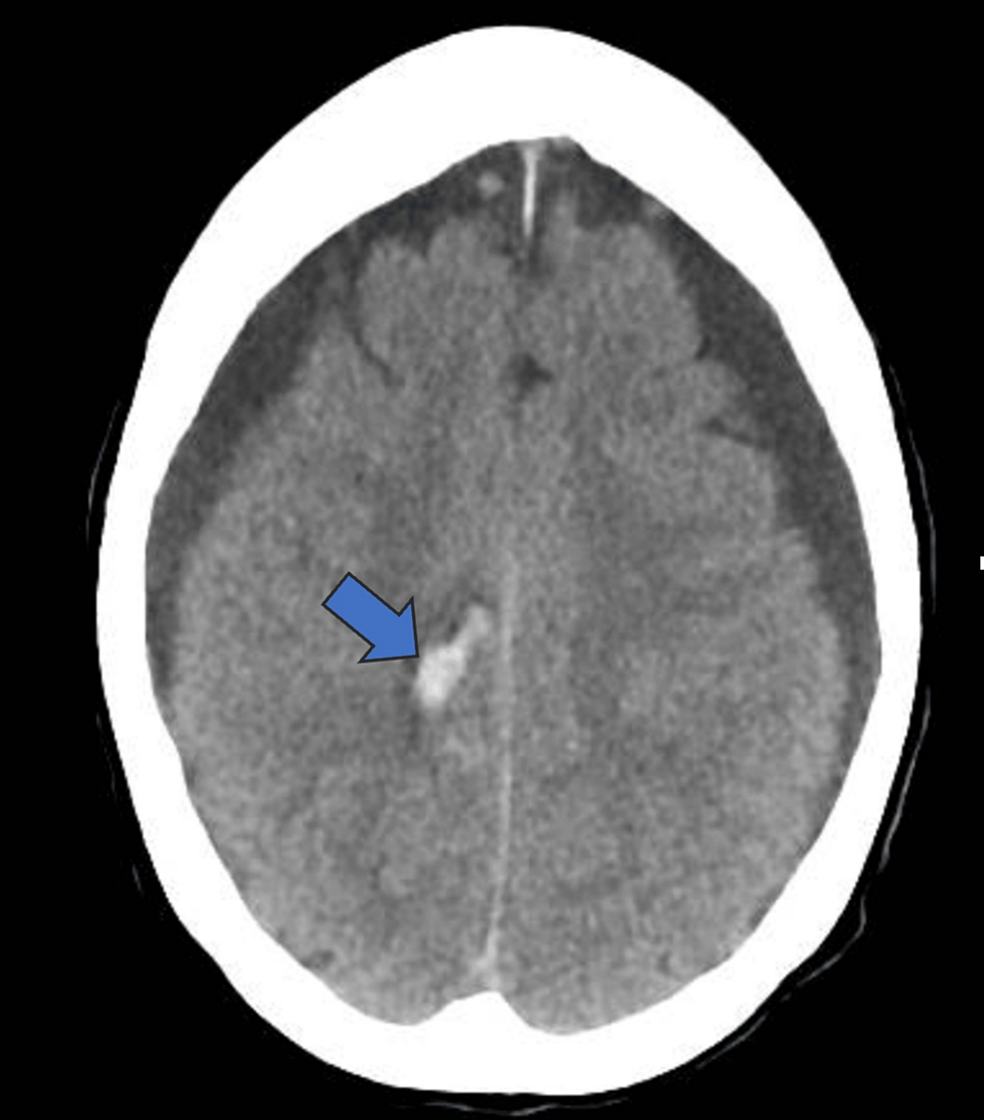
CT scan head showing intraparenchymal hemorrhage (arrow)
